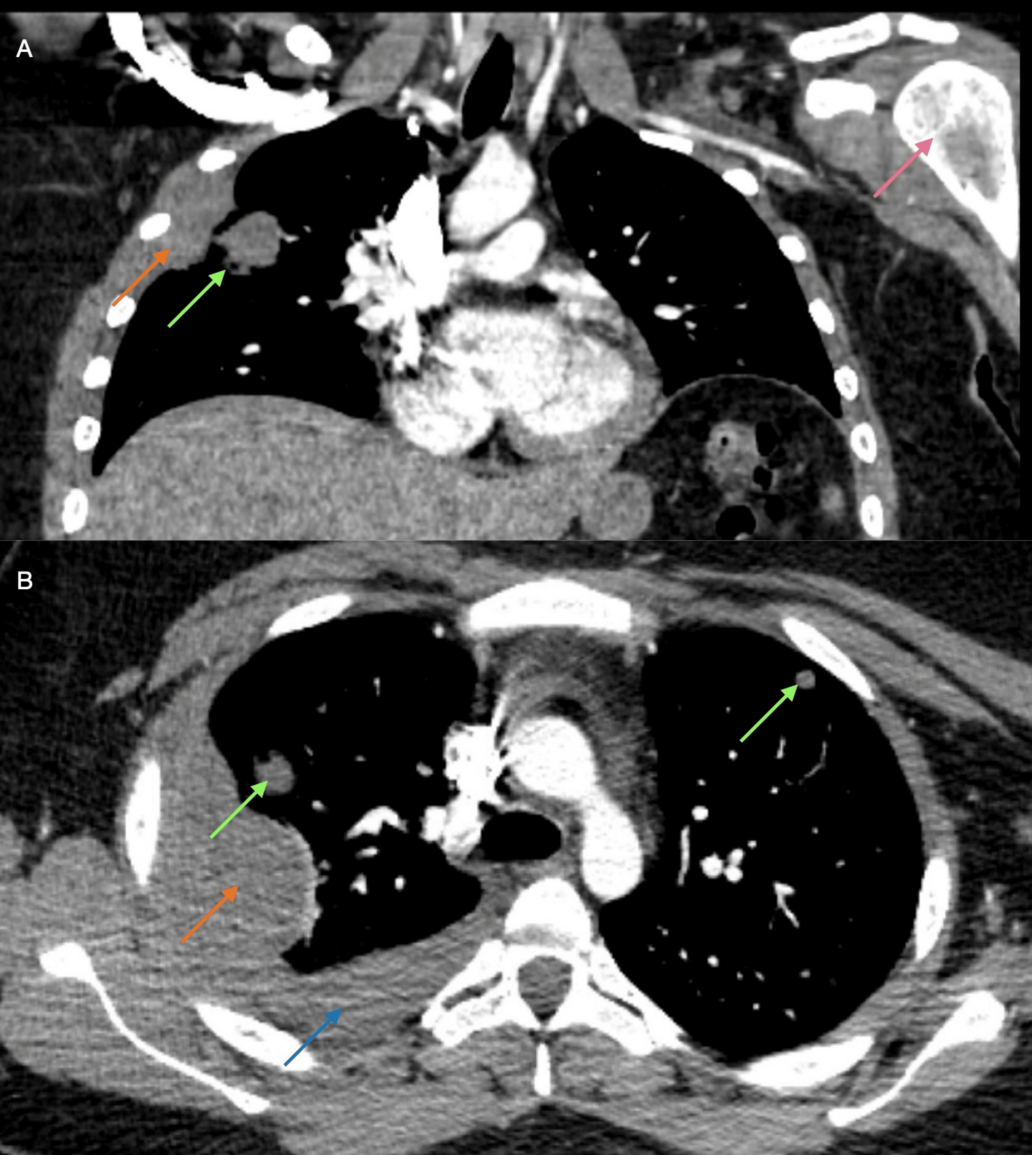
Selected coronal (A) and axial (B) views of CT chest with contrast obtained 3.5 months after symptom onset. Images show pulmonary metastases throughout both lungs (green arrows), pleural effusion (blue arrow), large pleural mass (orange arrows), and permeative appearance of the left proximal humerus (pink arrow). These findings are most consistent with metastatic disease.
